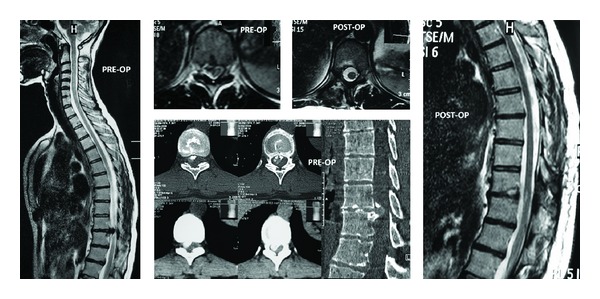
34-year-old female. In the neurological examination there was paraparesis in lower extremities (ASIA C). Cord compression of a thoracic 9-10 disc herniation (Case 10). Preoperative CT and MR images at the left side and postoperative images at the right side.
